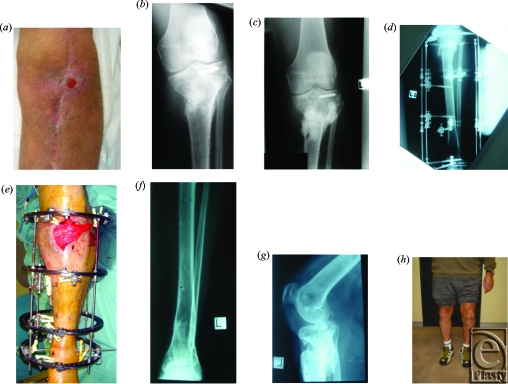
(*a*) Patient with chronic tibial OM and discharging sinus. (*b*) x-ray after removal of initial internal fixation and prior to debridement, demonstrating proximal tibial OM. (*c*) After debridement and insertion of antibiotic beads. (*d*) Osteotomy and application of Ilizarov frame. (*e*) Ilizarov frame and gastrocnemius flap. (*f*) Final result of distal tibial osteotomy site 10 months post-removal of Ilizarov frame. (*g*) Final result of proximal tibial site after bone grafting and removal of Ilizarov frame. (*h*) The final clinical result.
